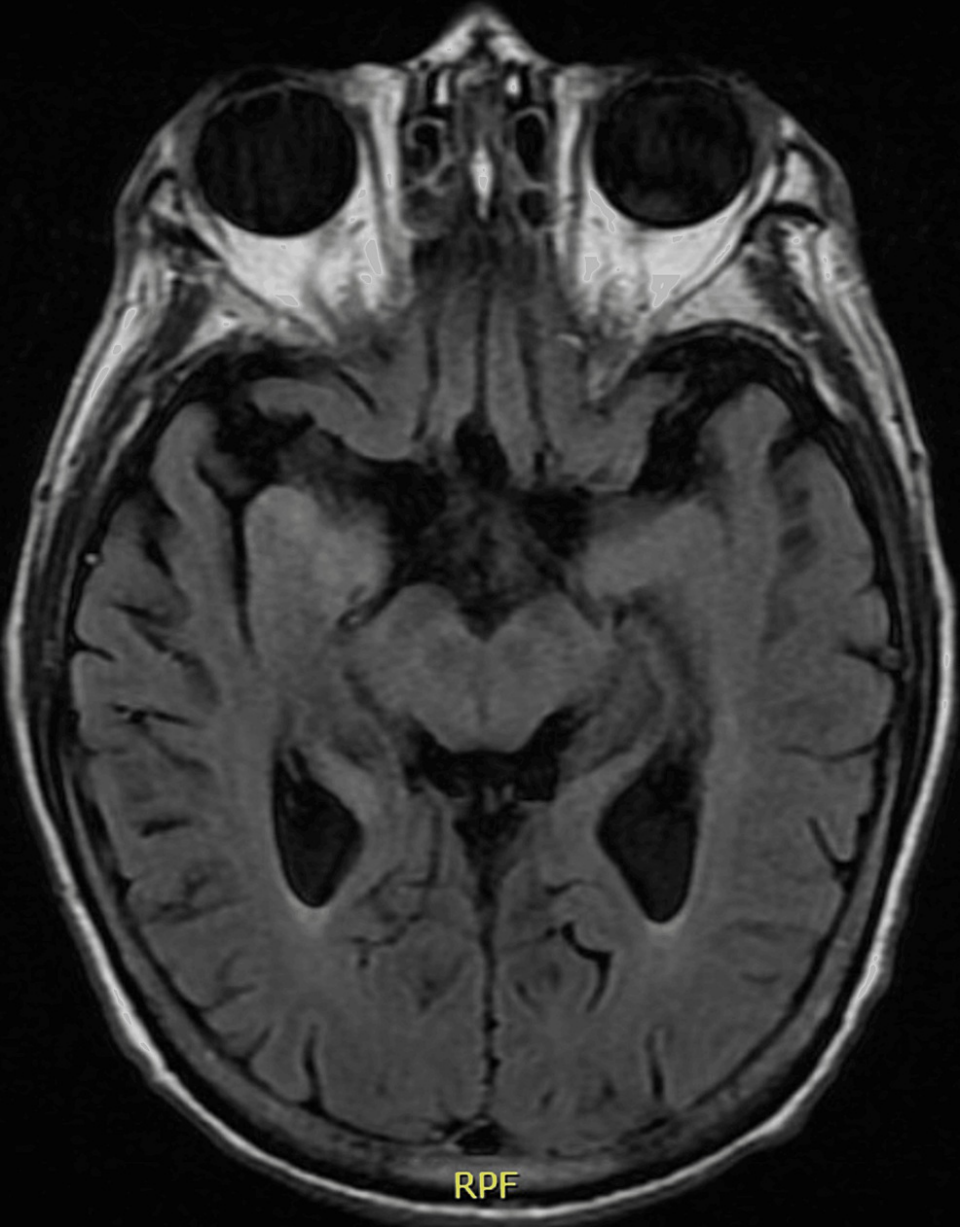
Brain magnetic resonance imaging Increased signal on fluid-attenuated inversion recovery imaging in the mesial right temporal lobe concerning right-sided limbic encephalitis.
